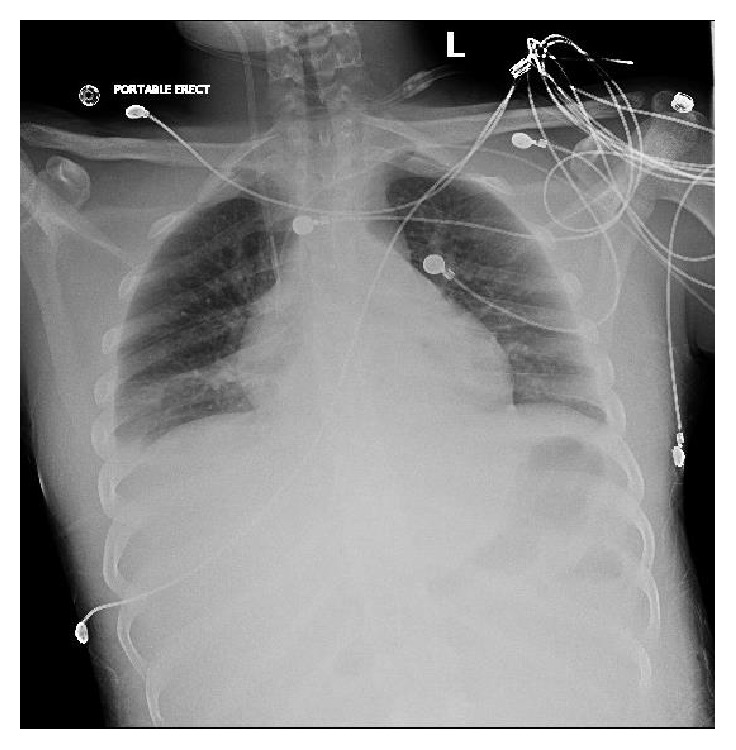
Chest X-ray AP view showing catheter projected over right atrium and superior IVC.
